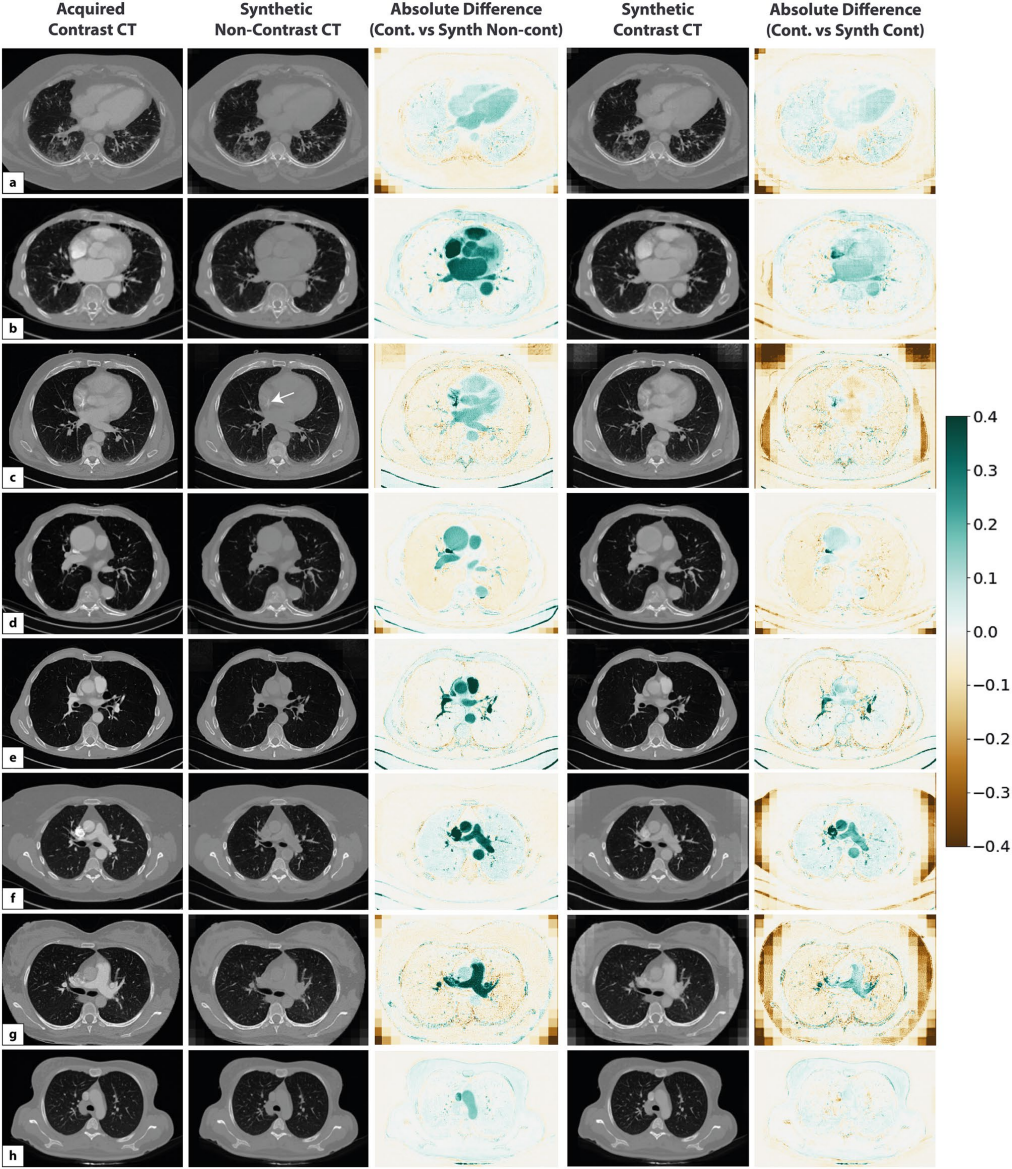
Results of cycle-GAN training on eight example test cases (a–h) for contrast to non-contrast CT synthesis. The absolute intensity difference maps show the scaled intensity differences between normalized acquired contrast/synthetic non-contrast and synthetic contrast/acquired contrast CTs (intensity range: −400, 400HU; normalized intensity range: −4.0, 4.0). The framework successfully removed and predicted contrast on test chest CTs (e.g. a,d,h). Contrast regions within synthetic contrast images were mainly removed from pulmonary arteries, with some cases showing intensity differences due to variable contrast agent injection timepoints (e.g. b,e,f,g). The white arrow represents structures where hypo-intensity remains on chest CTs after synthesis (e.g. c).
